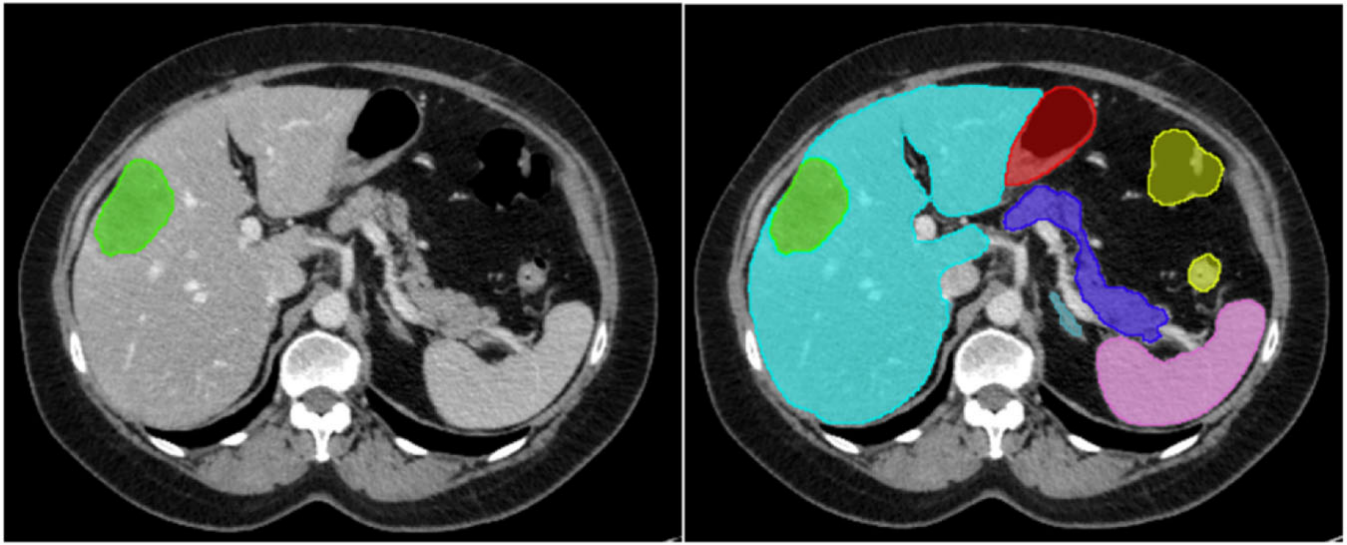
Example of a manually segmented portal venous phase axial computed tomography scan performed by a trio of radiologists and combined using the STAPLE algorithm. Green = CRLM, turquoise = liver, pink = spleen, dark blue = pancreas, light blue = adrenal glands, red = stomach, yellow = colon
